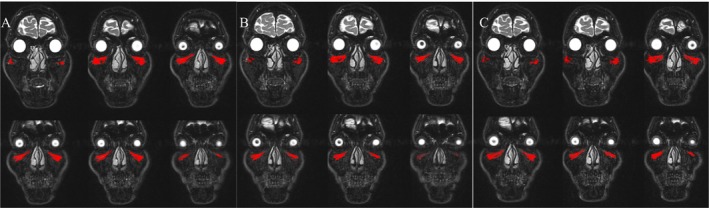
The coronal T2‐weighted fat‐saturated 3‐T MRI scan of a male subject, age 27 years, achieved a 0‐point improvement on the AIHS following treatment with Hearty. Red highlights indicates the signal of volume enhancement in the infraorbital region. (A) 1 weeks after injection, (B) 1 months after injection, and (C) 3 months after injection.
